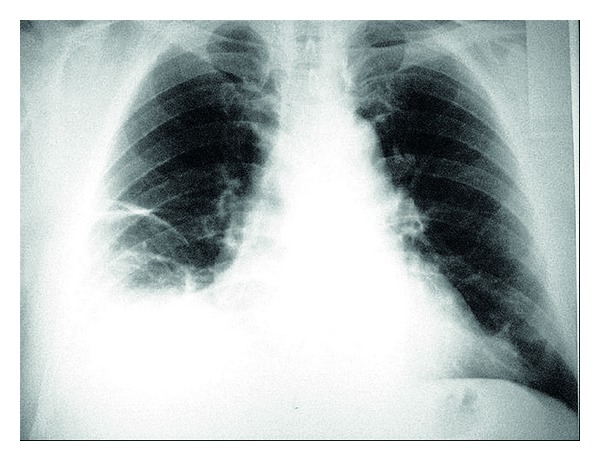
Chest radiography showing pleural effusion with linear atelectasis on the right lung.
